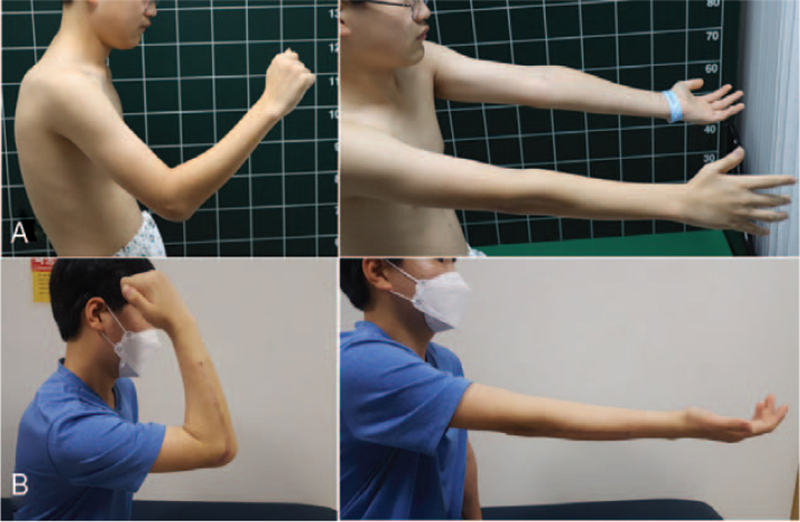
Radiographs taken at 2 yrs following the surgery. At the last follow-up, the delayed RHD associated with malunion of the radial shaft was corrected. RHD = radial head dislocation.
